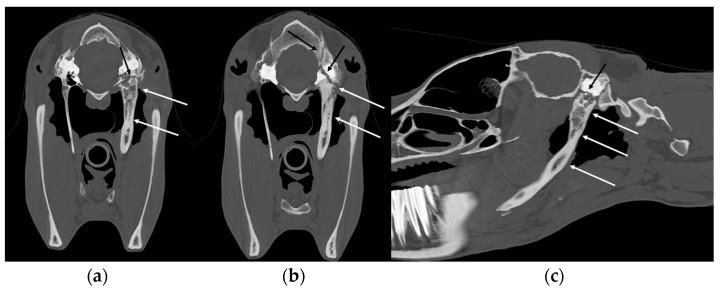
Transverse (a,b) and sagittal (c) CT images (bone window) of a pony with temporohyoid osteoarthropathy. Right is to the left. New bone formation and ankylosis can be observed at the level of the left temporohyoid joint associated with severe thickening of the left stylohyoid bone (white arrows). Several associated fracture lines are present through the left temporal bone (black arrows). Images courtesy of Dr Mickaël Robert.
